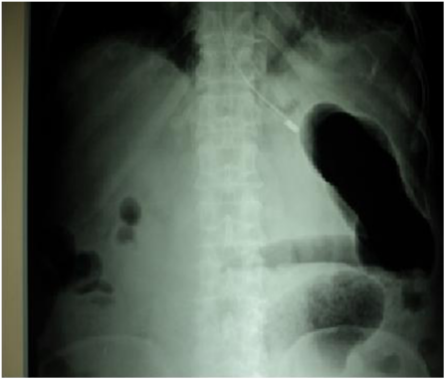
Upright abdominal plain film showing air-fluid level in dilated gut loops.
